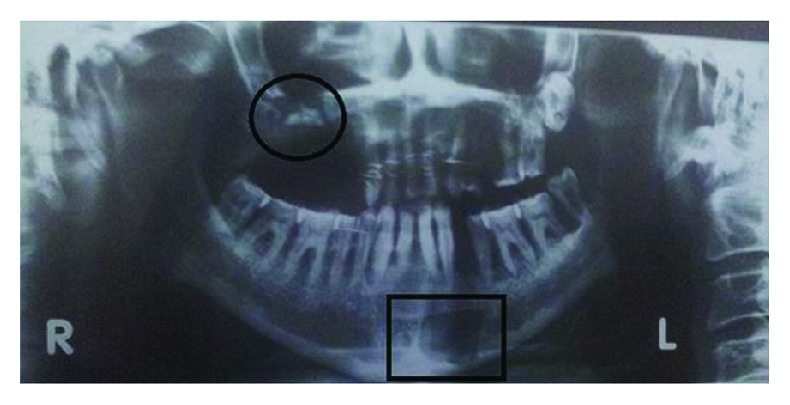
Radiograph showing chin graft well in place.
